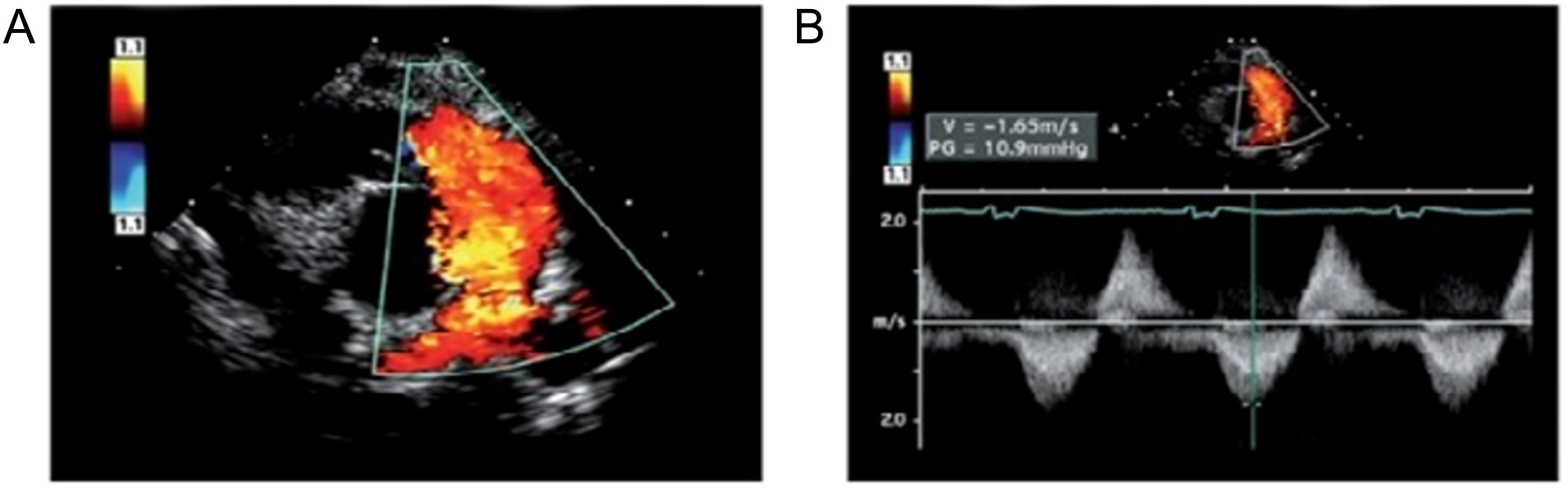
Echocardiography of severe pulmonary regurgitation in a patient
